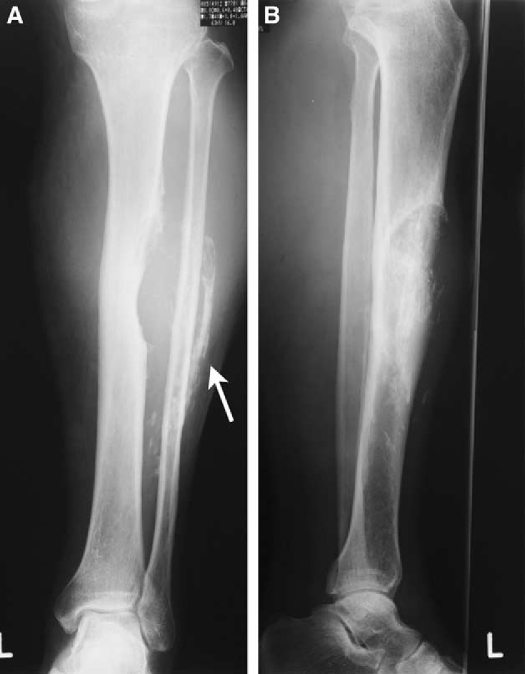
Plain radiographs of the left leg revealed a soft tissue mass in the anterolateral part of the leg with extensive amorphous calcifications (arrow).
